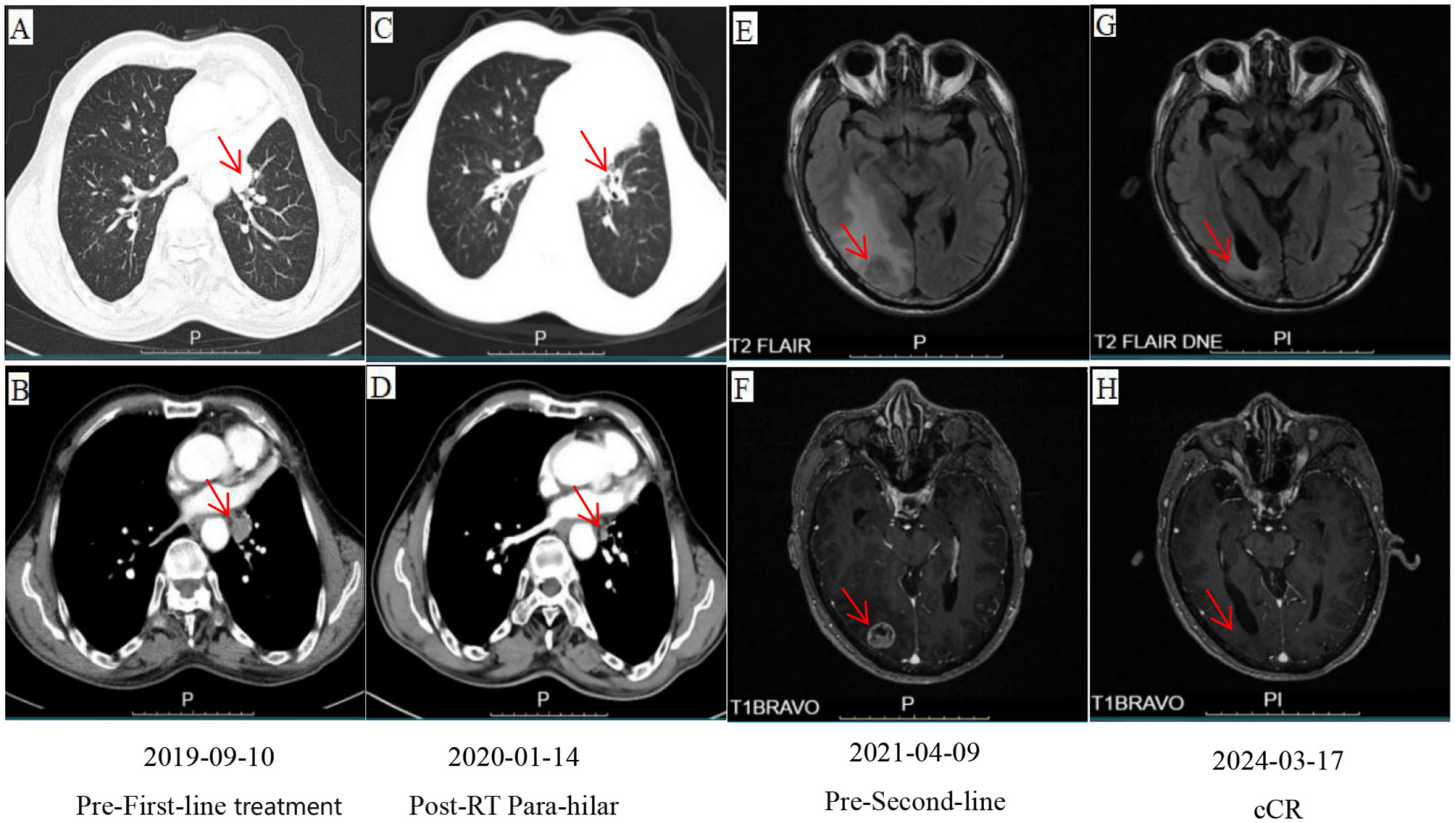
(A, B) CT images of the lung window (A) and the mediastinal window (B) on September 10, 2019; (C, D) CT images of the lung window (C) and the mediastinal window (D) on January 14, 2020; (E, F) MRI images of T2 FLAIR (E) and T1 BRAVO (F) on April 9, 2021; (G, H) MRI images of T2 FLAIR (G) and T1 BRAVO (H) on March 17, 2024.
